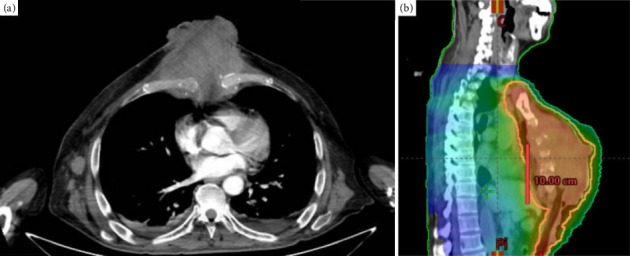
Imaging. (a) Transversal CT scan of the thorax. (b) Sagittal CT scan of the thorax showing planned radiotherapy target areas. *Source: Original work.*
